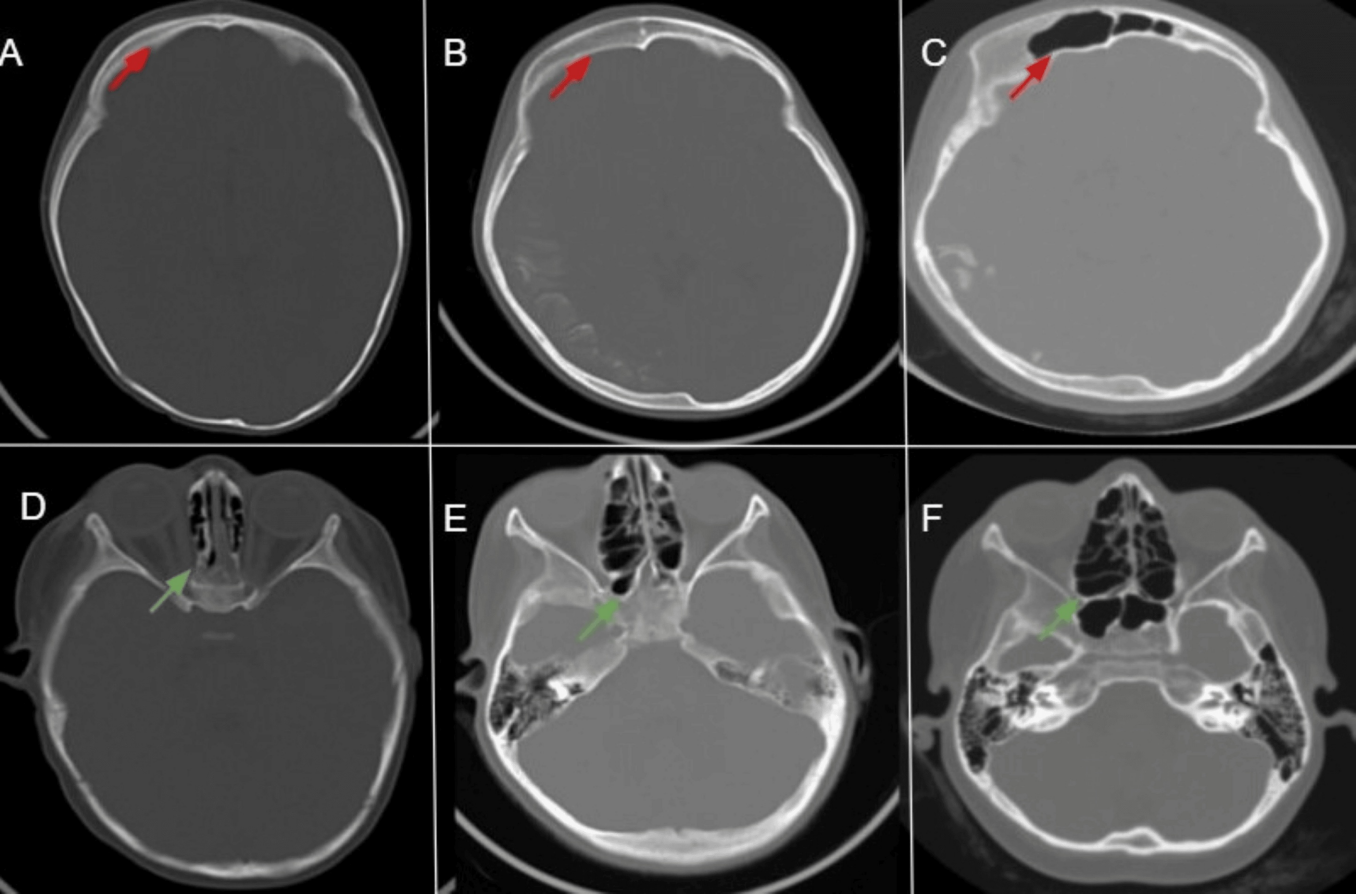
Axial CT bone window images Axial CT bone window images (A–C) demonstrate frontal sinus pneumatization with right-sided enlargement (red arrows) at one, six, and 11 years of age, respectively. Images (D–F) show ethmoidal and sphenoidal sinus pneumatization with right-sided enlargement (green arrows)at one, six, and 11 years of age, respectively.
